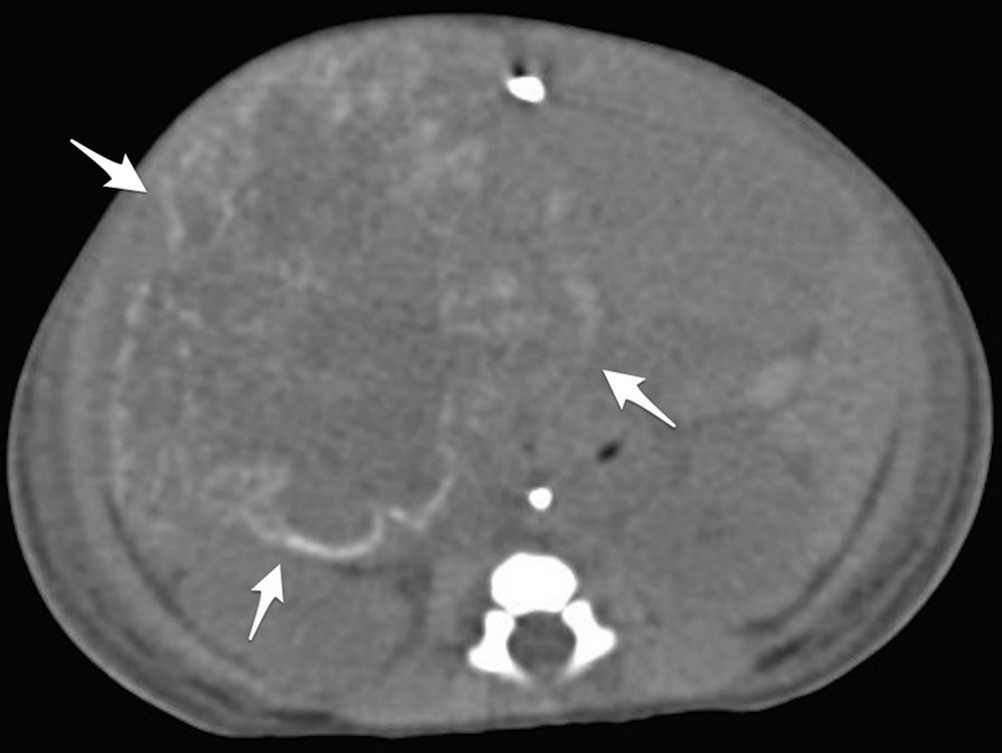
Non-enhanced CT scan showed predominantly peripheral calcifications (arrows).
